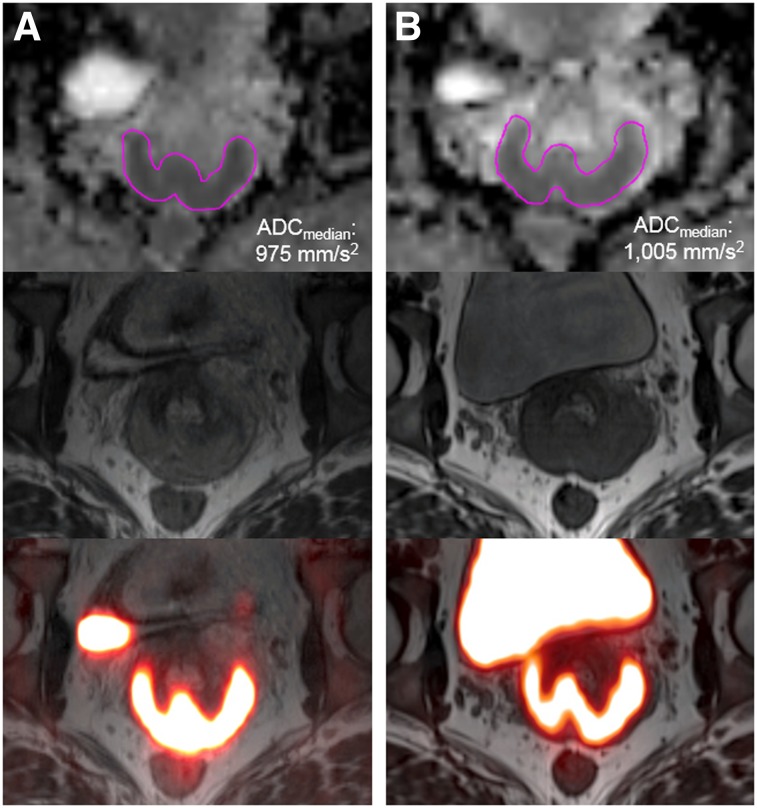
ADC_median_ repeatability from ADC map analysis. A 33-y-old woman with biopsy-proven squamous cell carcinoma of cervix was imaged with 48 h between session 1 (A) and session 2 (B). Transaxial ADC maps with manual contours (pink), T2-weighted MR images, and fused PET/MR images are shown from top to bottom for each imaging session. ADC_median_ values for sessions 1 and 2 were similar.
